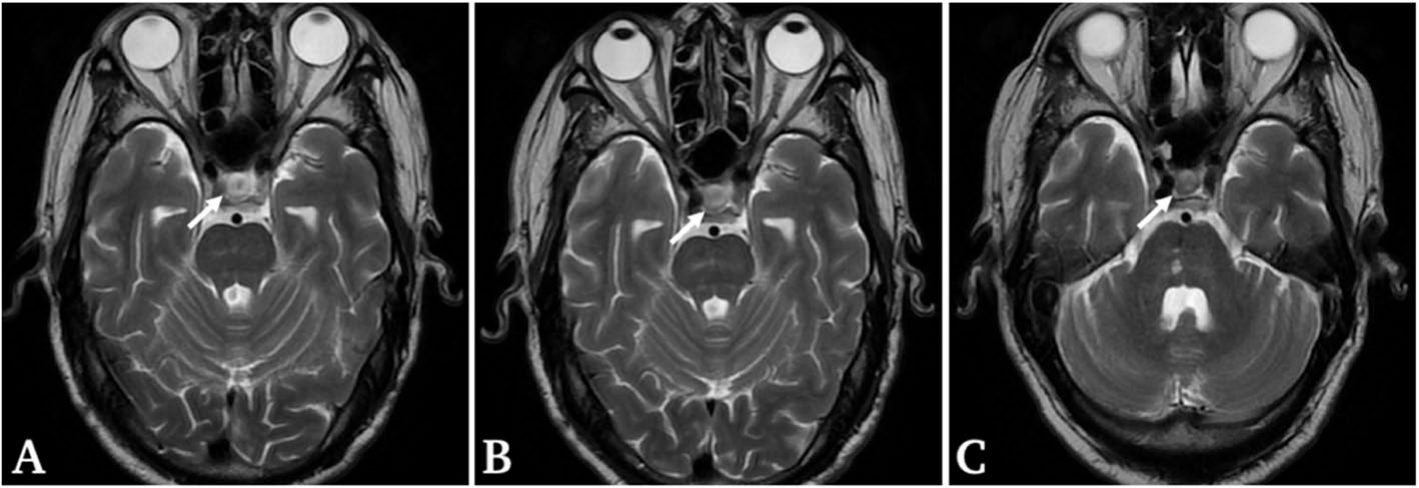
Magnetic resonance imaging (MRI) scans. A Brain MRI scans obtained before the diagnosis of isolated adrenocorticotropin deficiency (August 2020). B, C Brain MRI scans obtained at and after the diagnosis of IAD, respectively (September and November 2020). A-C all MRI scans showing a normal size of pituitary gland and no obvious enhancement was observed (white arrows)
